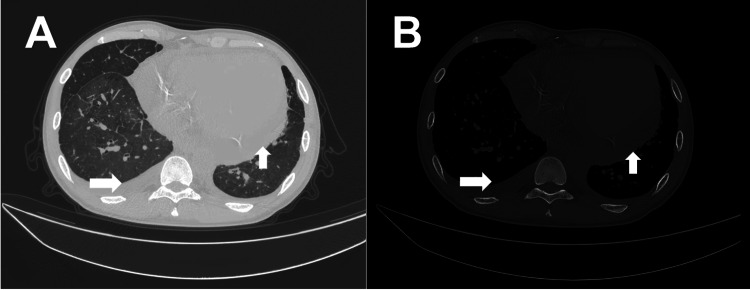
Multiserosal Effusions With Cardiopulmonary Abnormalities on Chest CT (A) Chest CT in lung window and (B) mediastinal window demonstrating pulmonary edema and pneumonia, bilateral pleural effusion, left heart enlargement, pericardial effusion, and ascites (representing multiple serous cavity effusions). CT: computed tomography
